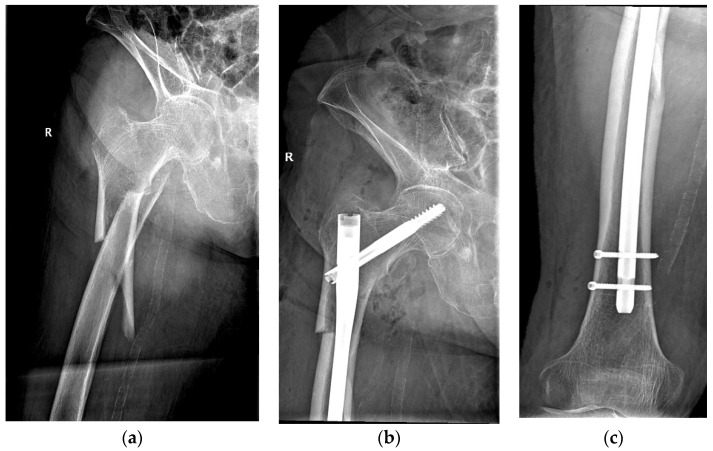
An 84-year-old patient diagnosed with a subtrochanteric fracture after a fall from the same height treated with CRIF with long Gamma Nail fixation: (a) preoperative radiograph; (b,c) postoperative radiographs.
